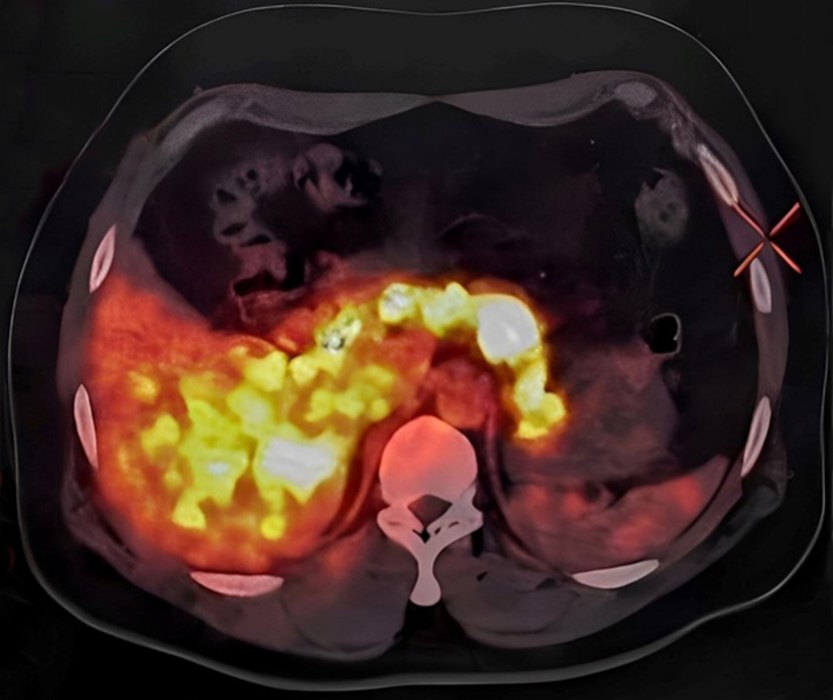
Ga 68 Exendin PET/CT: Showed diffuse uptake in the body and tail of pancreas.
